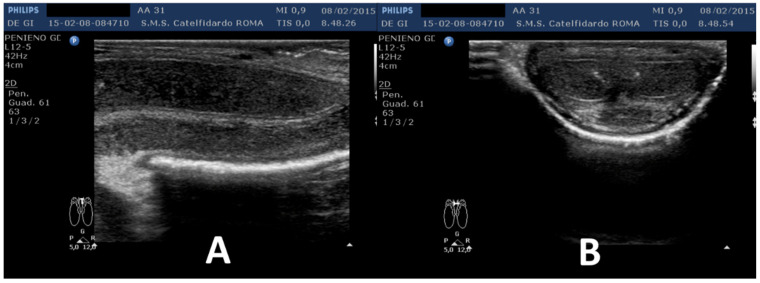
Ultrasonography of the penis after the first treatment cycle. (A) Longitudinal view, (B) transverse view.
